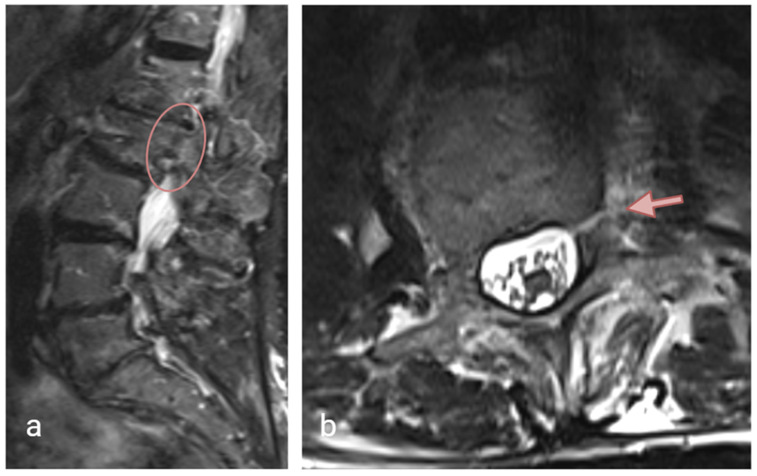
MRI at 3 months; (a) sagittal T2/STIR sequence and (b) axial T2W images demonstrate a visible fracture line at the left L2 pedicle.
